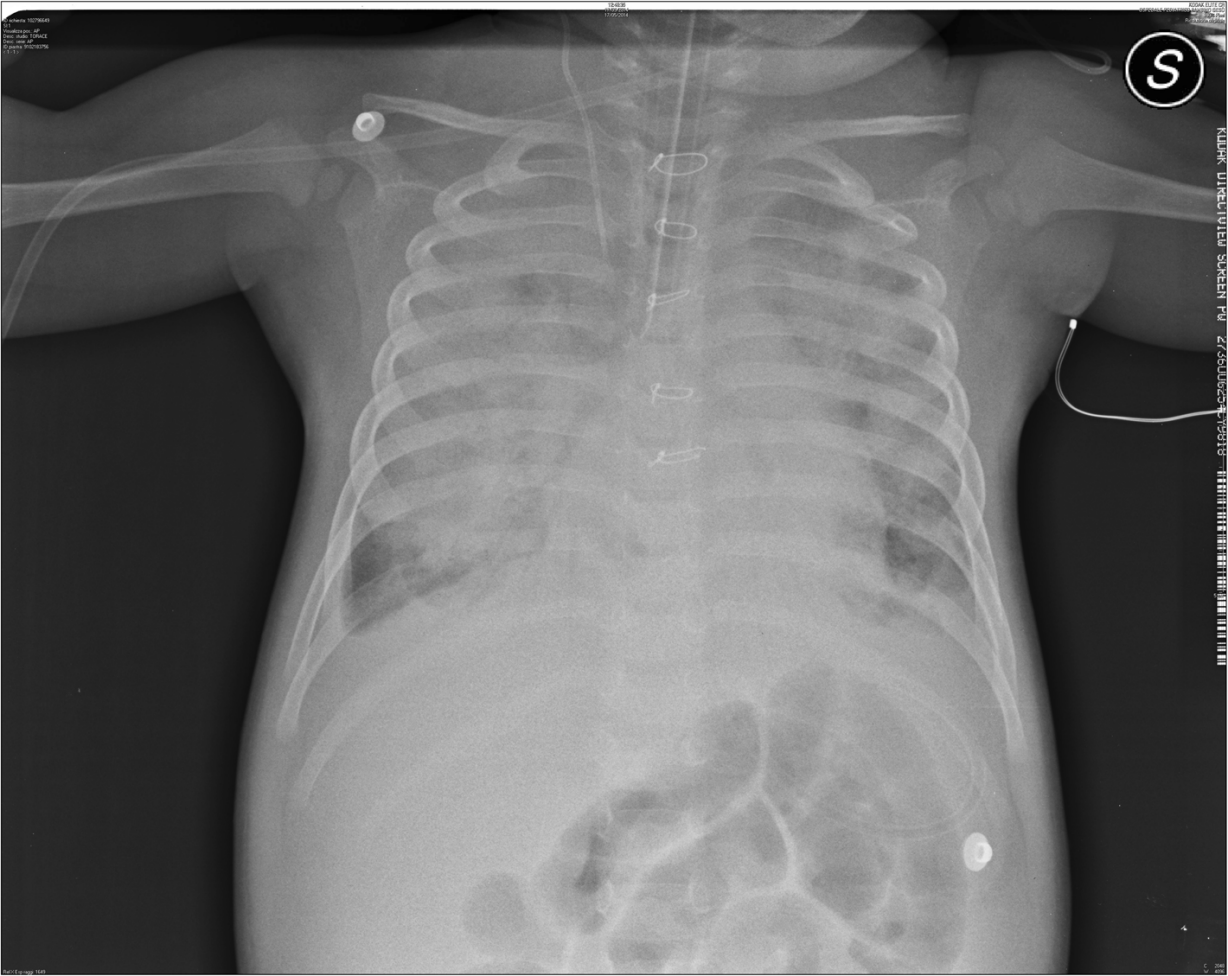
Chest X-Ray: multiple foci of parenchymal spread to both lungs and pleural effusion obliterating share of the breast-phrenic cost
